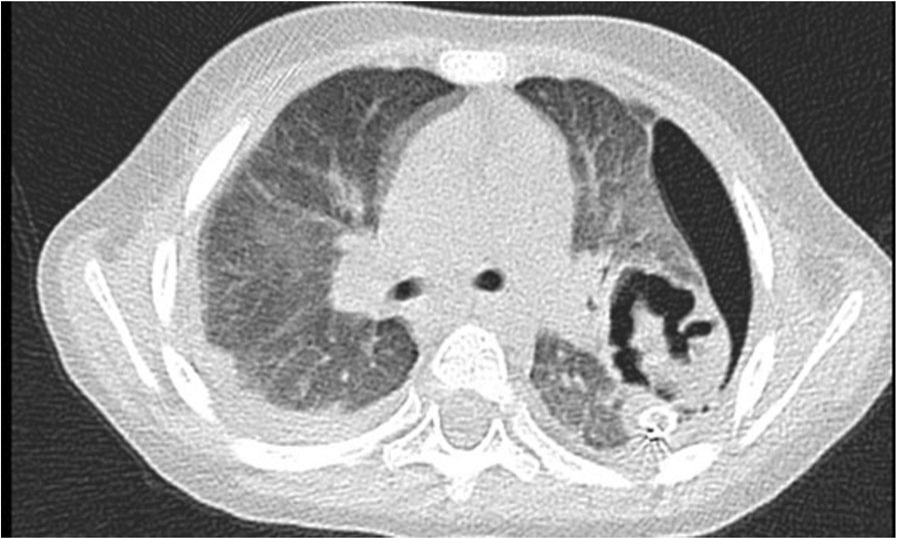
Axial computed tomography image of the patient before operation
